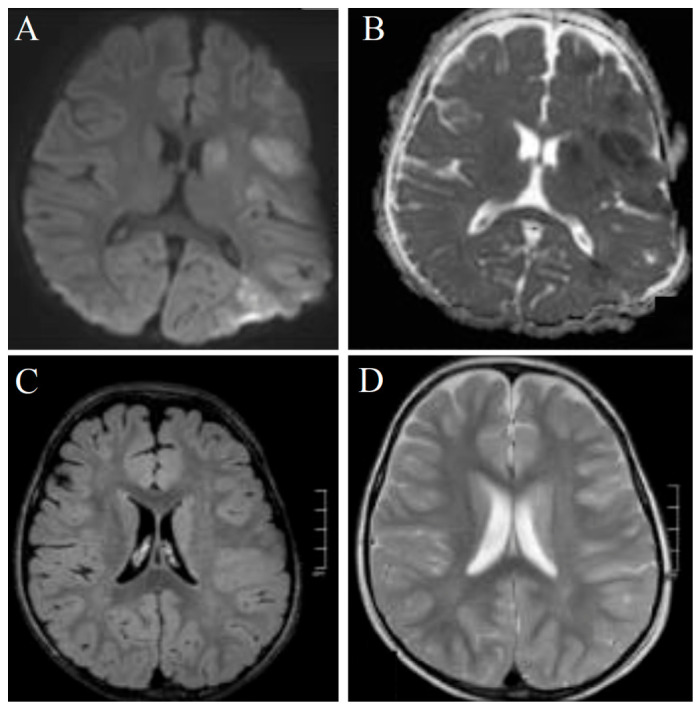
Brain MRI demonstrating a hyperacute ischemic infarction in the left middle cerebral artery (MCA) territory. Diffusion-weighted imaging (DWI) shows a focal hyperintense lesion in the left cerebral hemisphere (A) with corresponding hypointensity on the apparent diffusion coefficient (ADC) map (B), confirming restricted diffusion. No corresponding signal abnormality is observed on FLAIR (C) or T2-weighted imaging (D), consistent with a hyperacute stage of infarction before the development of conventional MRI changes.
